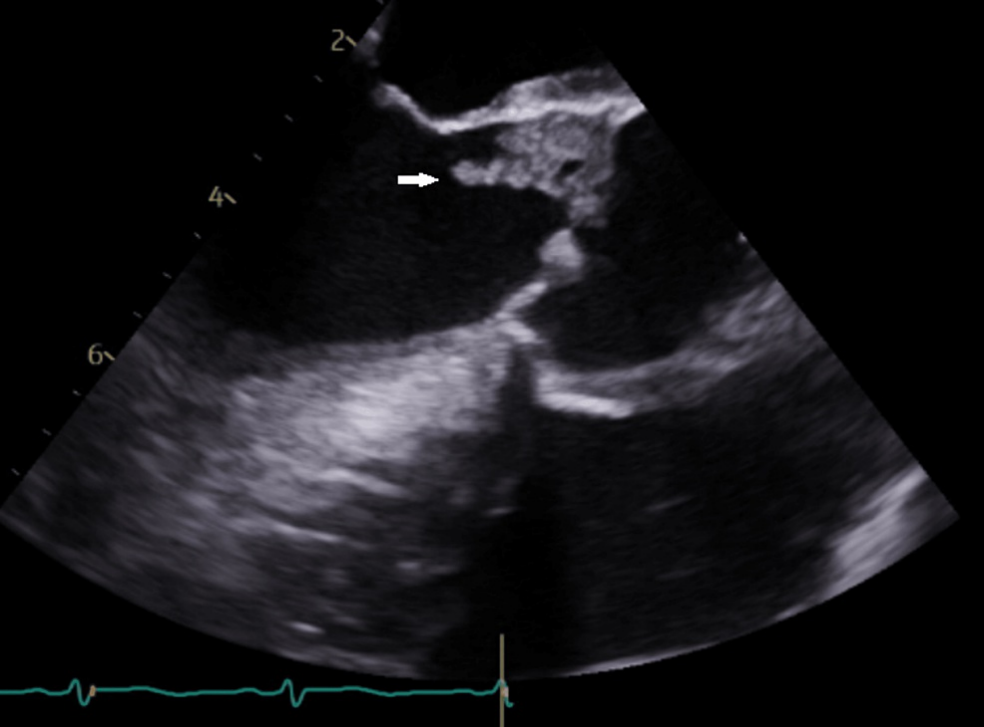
TEE showed the presence of a large vegetation. Large vegetation had both sessile as well as a mobile elongated piece measuring about 2 cm and was attached to the noncoronary cusp of the aortic valve (arrow). TEE: transesophageal echocardiogram
